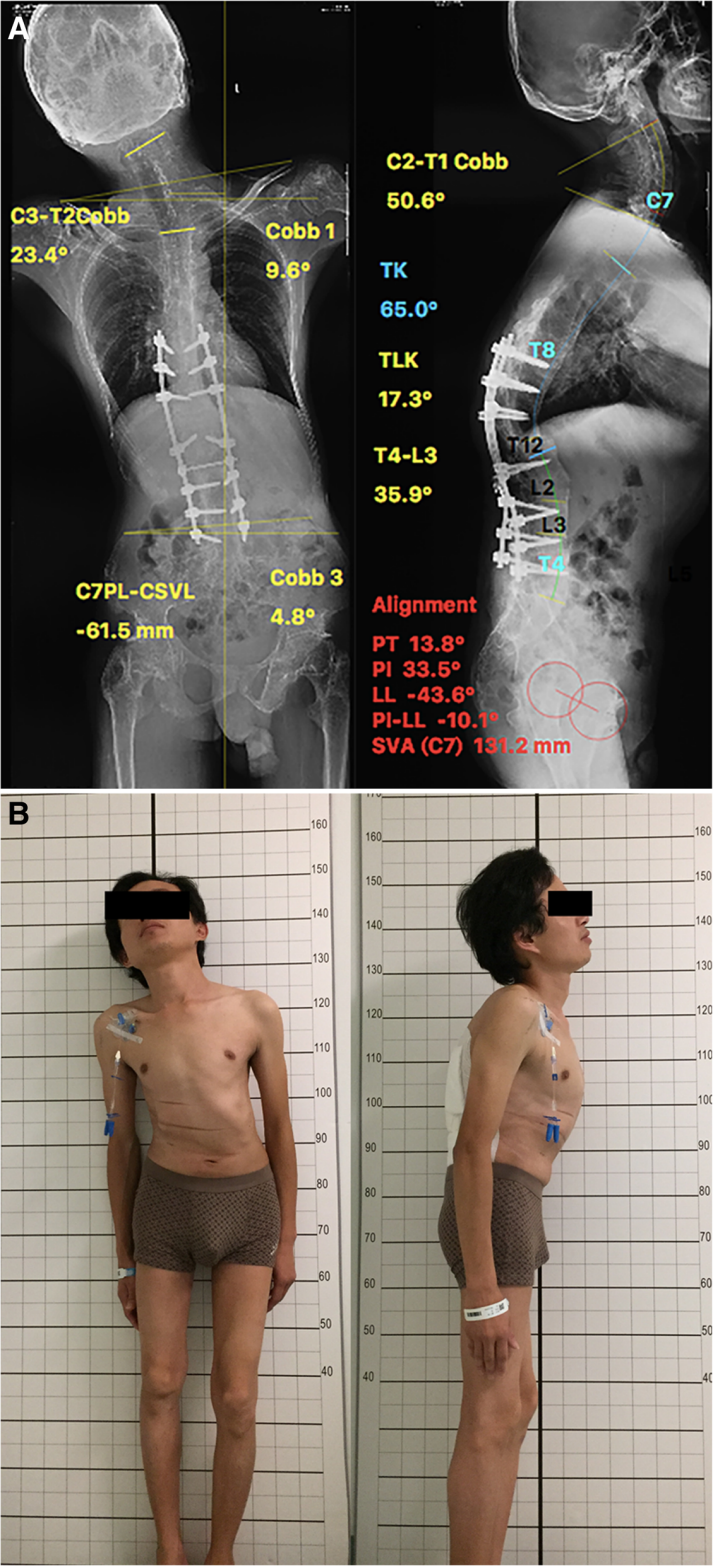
a Postoperative standing X-ray of the AS patient after interrupted two-level VCD at T12 and L2. The TK, PT, PI, and SVA were 65.0°, 13.8°, 33.5°, and 131.2 mm, respectively. The total kyphosis of the spine (T4–L3) was 35.9°. The Cobb angle of C2–T1 in the sagittal plane was 50.6°. The Cobb angle of C3–T2 in the coronal plane was 23.4°. b Postoperative photographs of the first stage showing adequate correction of kyphoscoliosis. He cannot see anything within 3 m. His CBVA was − 21.7°
